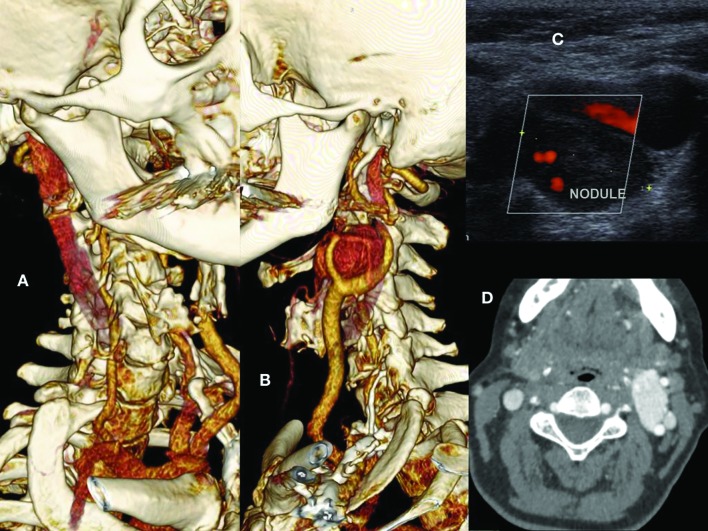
(A) 3D angiotomography reconstruction showing occlusion of the right carotid artery and vicarious vertebral artery; (B) 3D angiotomography reconstruction showing glomus tumor at the left carotid bifurcation; (C) Doppler ultrasonography showing a hypervascularized nodule between the left internal and external carotids; (D) Axial angiotomography slice showing hypervascularized glomus tumor on the left and carotid occlusion on the right.
